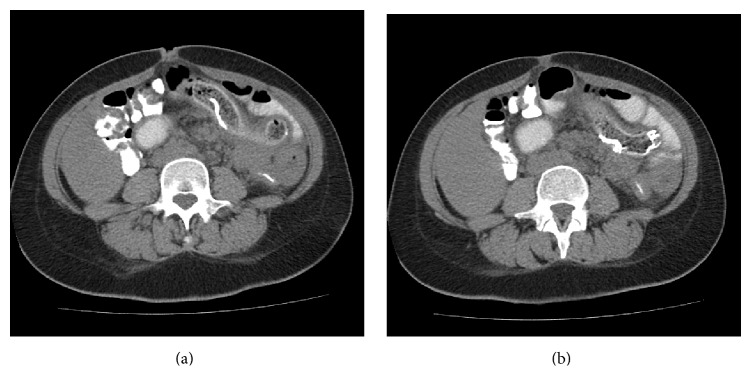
CT images revealing a calcified object inside bowel lumen.
